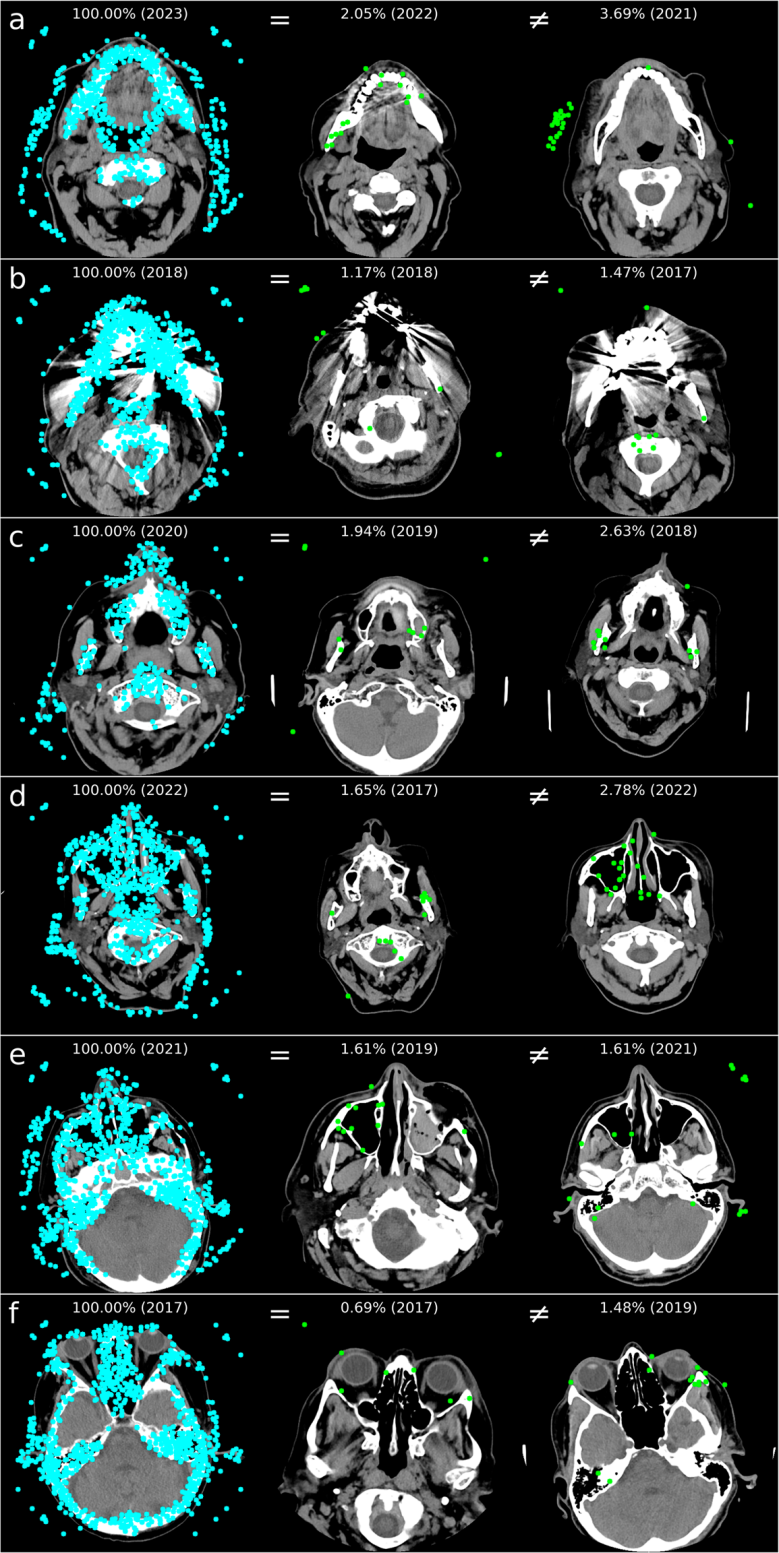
For unsuccessful identifications, examples of search images (left) for regions (a–f) and the best result (highest score) for the same identity (“=“, middle) and for different identities (“≠“, right) with the indication of the score in percentage and the image acquisition year in parentheses. Similar objects, like medical accessories (a) or CT tables, can result in a higher score for different individuals. Metal artifacts can also hinder identification (b). The various head positions during imaging of the search image and the reference image can complicate personal identification, as fewer matching points may be found (c–f)
